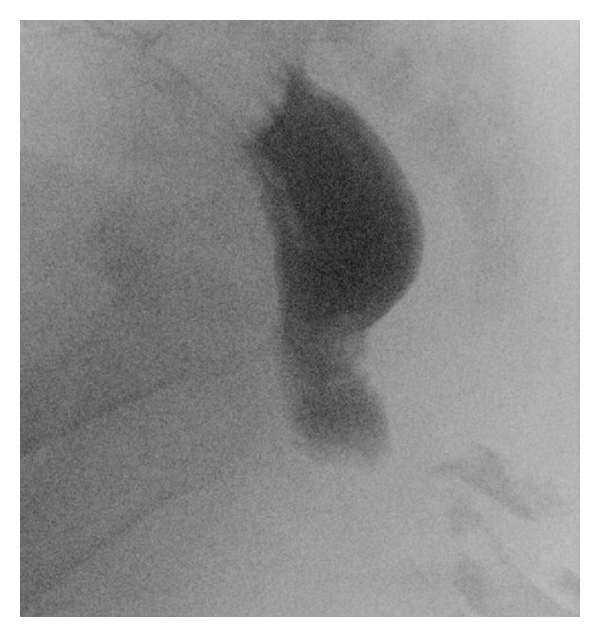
X-ray with contrast in the passive stoma. Before closure of the stoma, an open passage over the anastomosis was secured.
